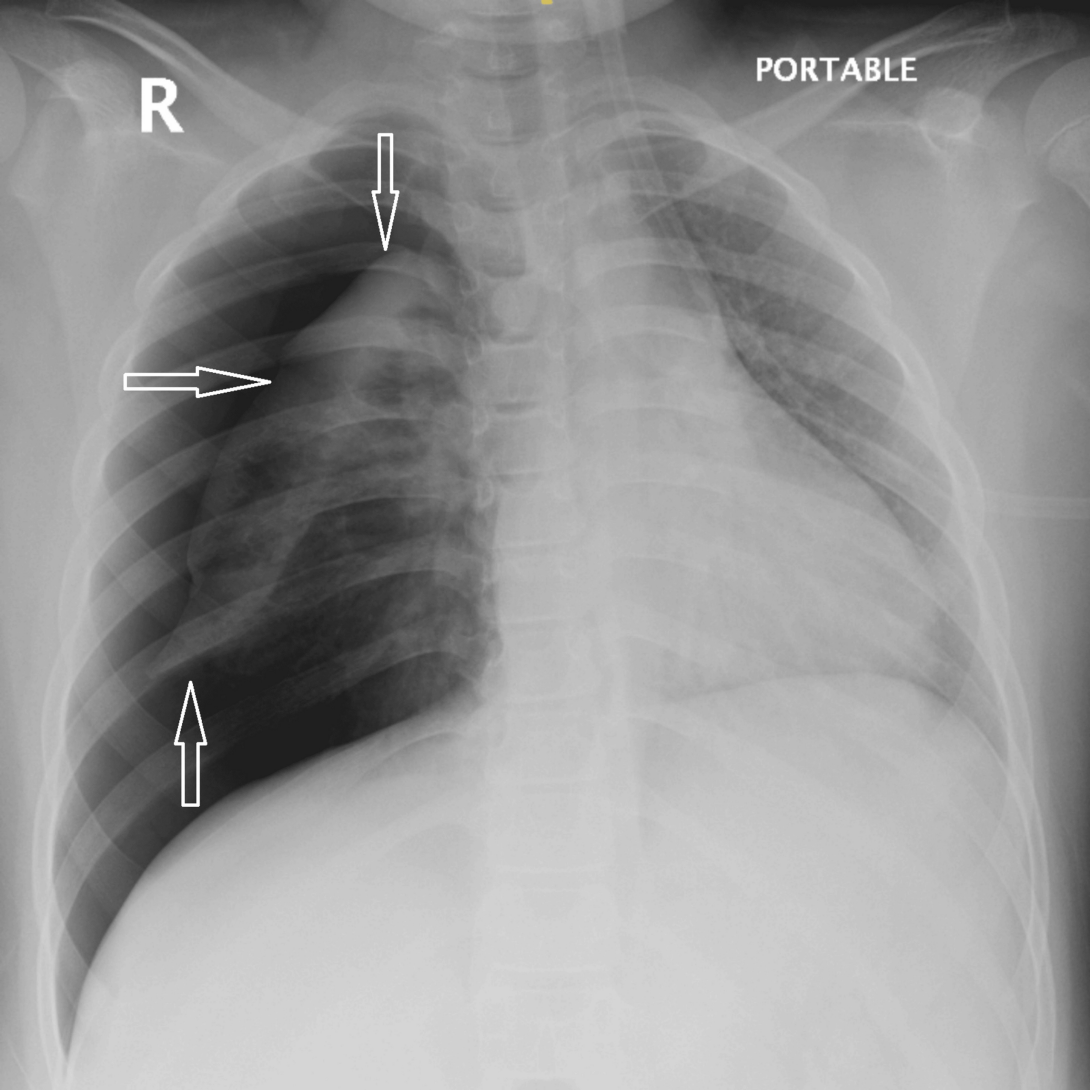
Chest radiograph of right-sided pneumothorax Chest X-ray demonstrating a marked right-sided pneumothorax with subtotal lung collapse. Arrows indicate the collapsed lung margin surrounded by the thin visceral pleura, and the pleural air space is clearly visible.
